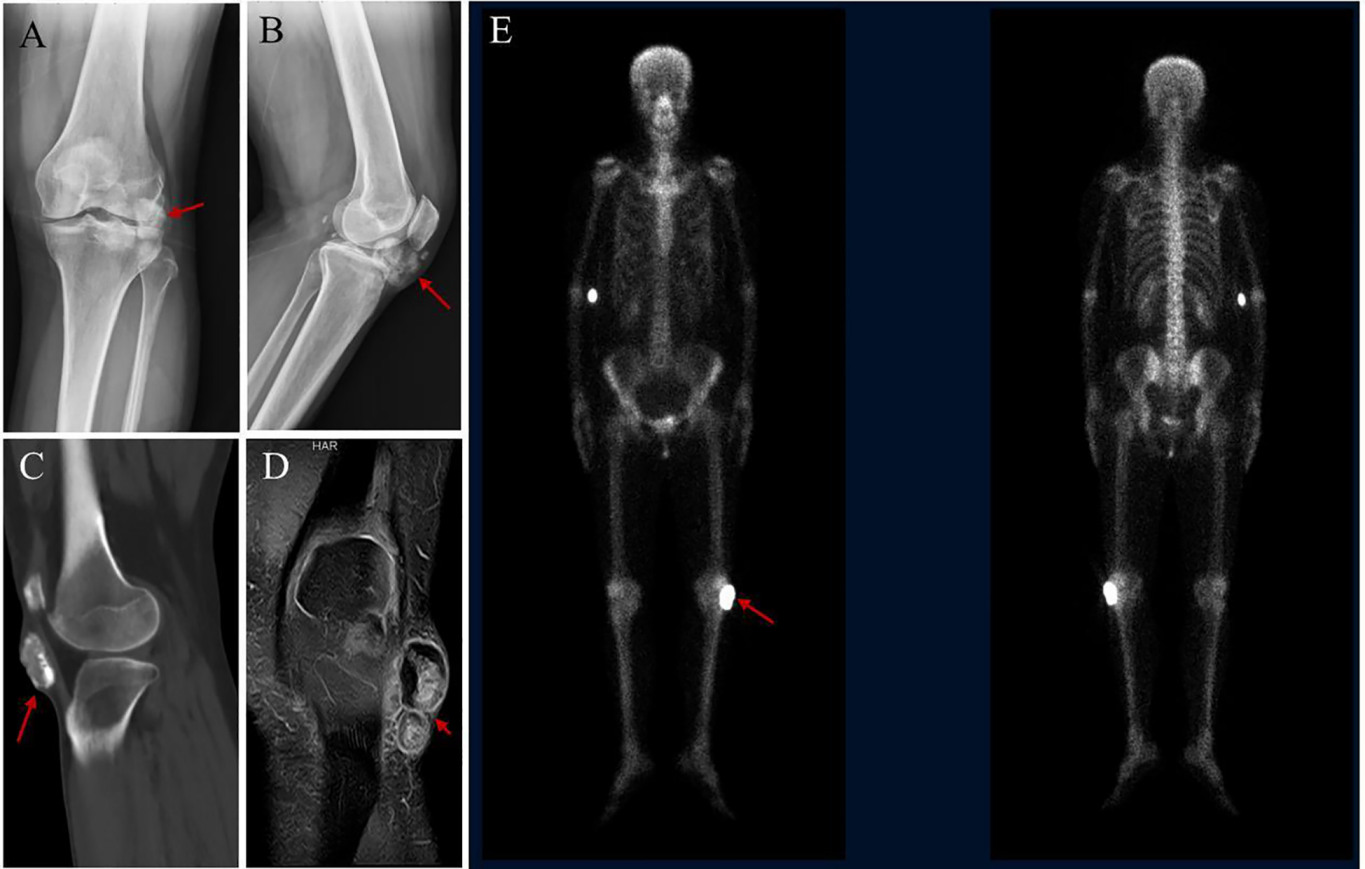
Radiological findings of the lesion. (A, B) X-ray shows nodular and flake-like high-density shadows under the left patella, approximately 5.4×4.5cm in size, with rough edges. (C) CT showed nodular high- density shadow in the subcutaneous tissue of the anterior inferior part of the left knee joint. (D) MRI showed a nodular short T2 signal shadow in the subcutaneous tissue of the anterior and inferior part of the left knee, with high signal intensity and low signal edge by fat suppression. (E) SPECT showed increased bone metabolism in the left knee joint region.
